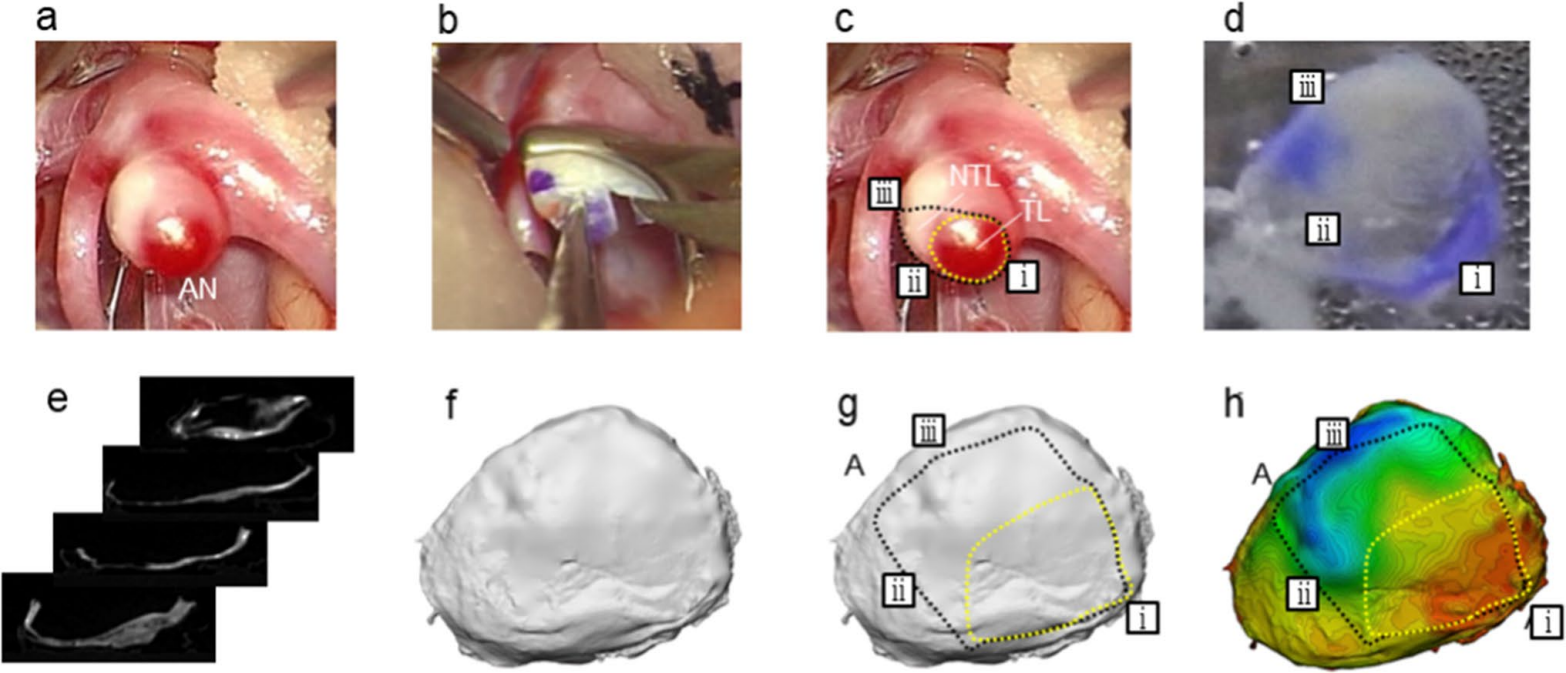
Experimental procedure for measuring three-dimensional aneurysmal wall thicknesses: a intraoperative appearance of an aneurysm (AN), b marking and harvesting, c wall-type classification, d harvesting of tissue, e tissue dehydration and micro-computed tomography measurement, f three-dimensional volume reconstruction, de-noising, and remeshing, g wall-type mapping and segmentation, and h three-dimensional wall-thickness distribution. The black and yellow dotted lines are explained in the caption of Fig. 1. The orientation of the three-dimensional model matches that of the intraoperative appearance before marking and harvesting
